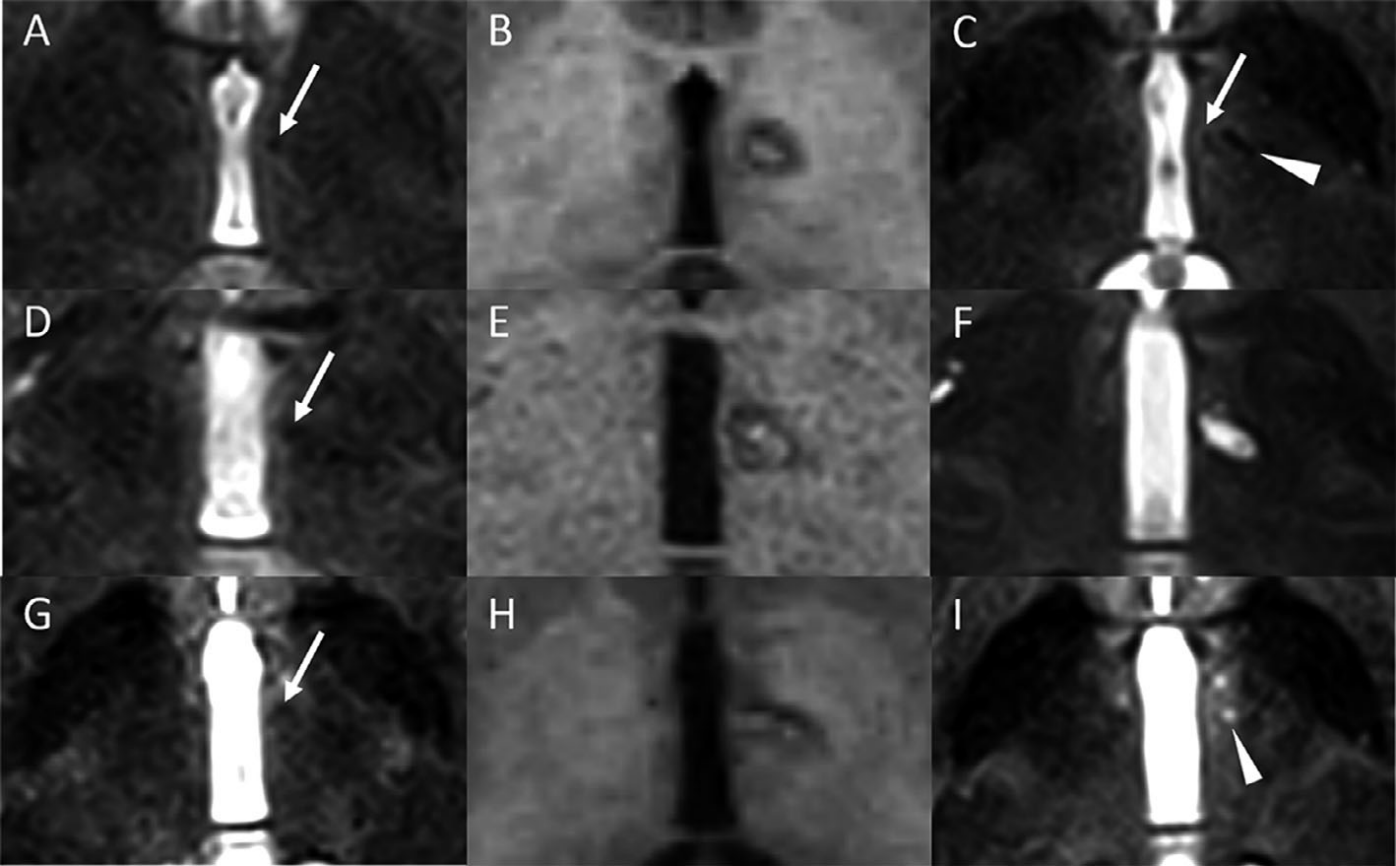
Pre‐ and postoperative MRI (day 0 and 3 months). (A–C) (successful case): Arrow showing left mammillothalamic tract (MTT) on the T2‐weighted MRI before the surgery. (A) Postoperative T1‐weighted MRI on the day of the surgery showing correct lesion location. (B) The 3‐month postoperative T2‐weighted MRI showing old lesion (arrowhead) and MTT (arrow). (C) D–F (hemorrhage case): Arrow showing left MTT on the T2‐weighted MRI before the surgery (D). Postoperative T1‐weighted MRI on the day of the surgery showing hemorrhage medially deviated. (E) The 3‐month postoperative T2‐MRI showing old scar involved MTT. (F) G–I (transient amnesia case): Arrow showing left MTT on the T2‐weighted MRI before the surgery. (G) Postoperative T1‐weighted MRI on the day of the surgery showing lesion medially deviated. (H) The 3‐month postoperative T2‐MRI showing old scar (arrowhead) and MTT is invisible (I).
